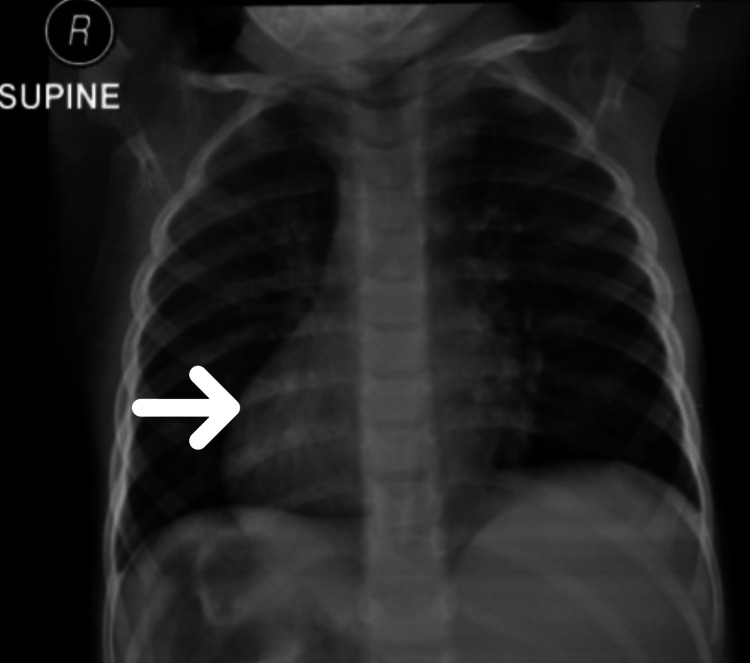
Chest X-ray (anteroposterior view) depicting dextrocardia (white arrow)
